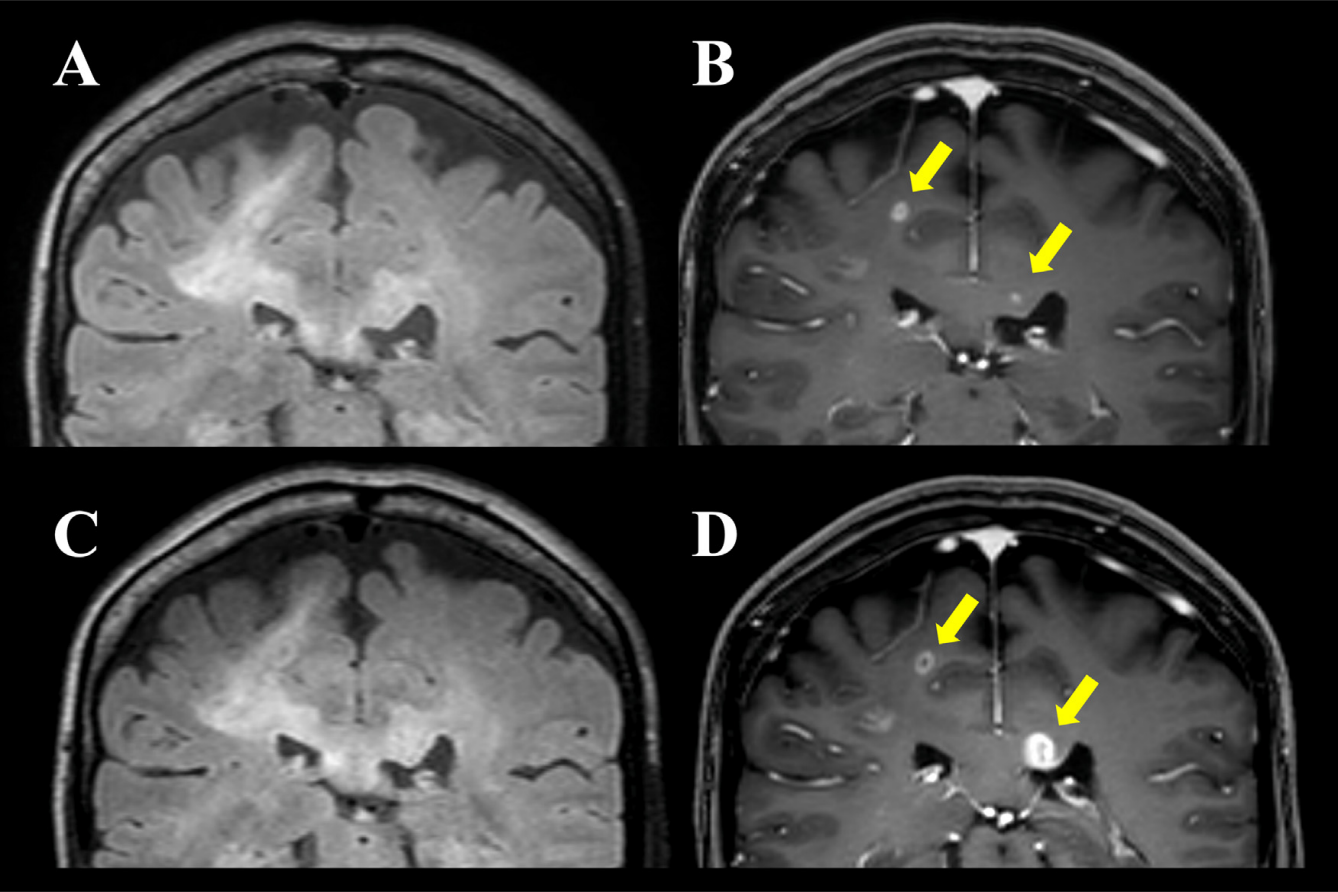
Brain MRI findings. Brain MRI in coronal view at initial evaluation at our hospital shows that the ill-defined high signal intensity area on FLAIR imaging (A) extends to the left side of the corpus callosum, with enhancement observed in the medial right precentral and postcentral gyri as well as the left corpus callosum on contrast-enhanced T1-weighted imaging (B, arrows). Follow-up MRI 2 months later shows no change in the ill-defined high signal intensity area on FLAIR imaging (C); however, enlargement of the enhancing lesions and ring-like enhancement are noted on contrast-enhanced T1-weighted imaging (D, arrows).
